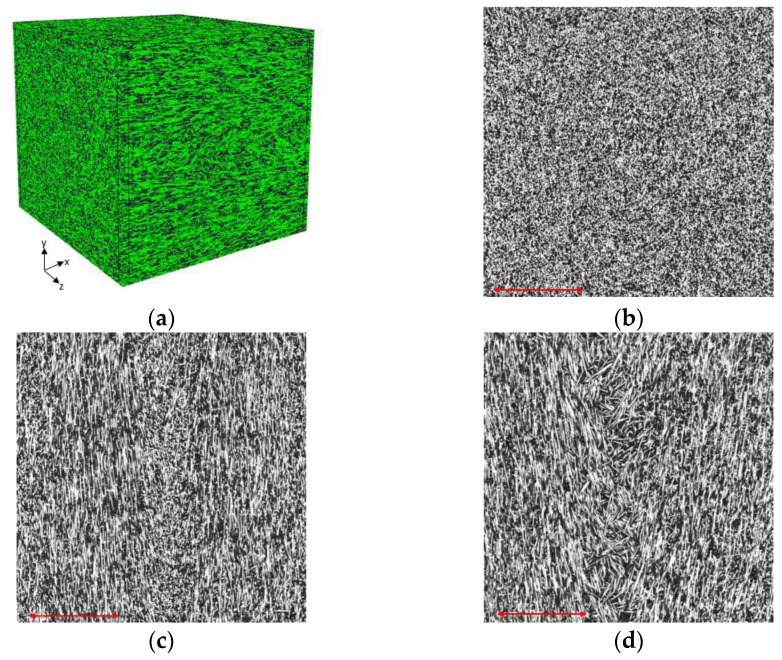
Miro-CT scans of the injection-moulded part: (a) a 3D view, (b) in y–z planes, (c) in x–z planes and (d) in x–y planes. (The scale bar in each figure shows a distance of 1 mm).
